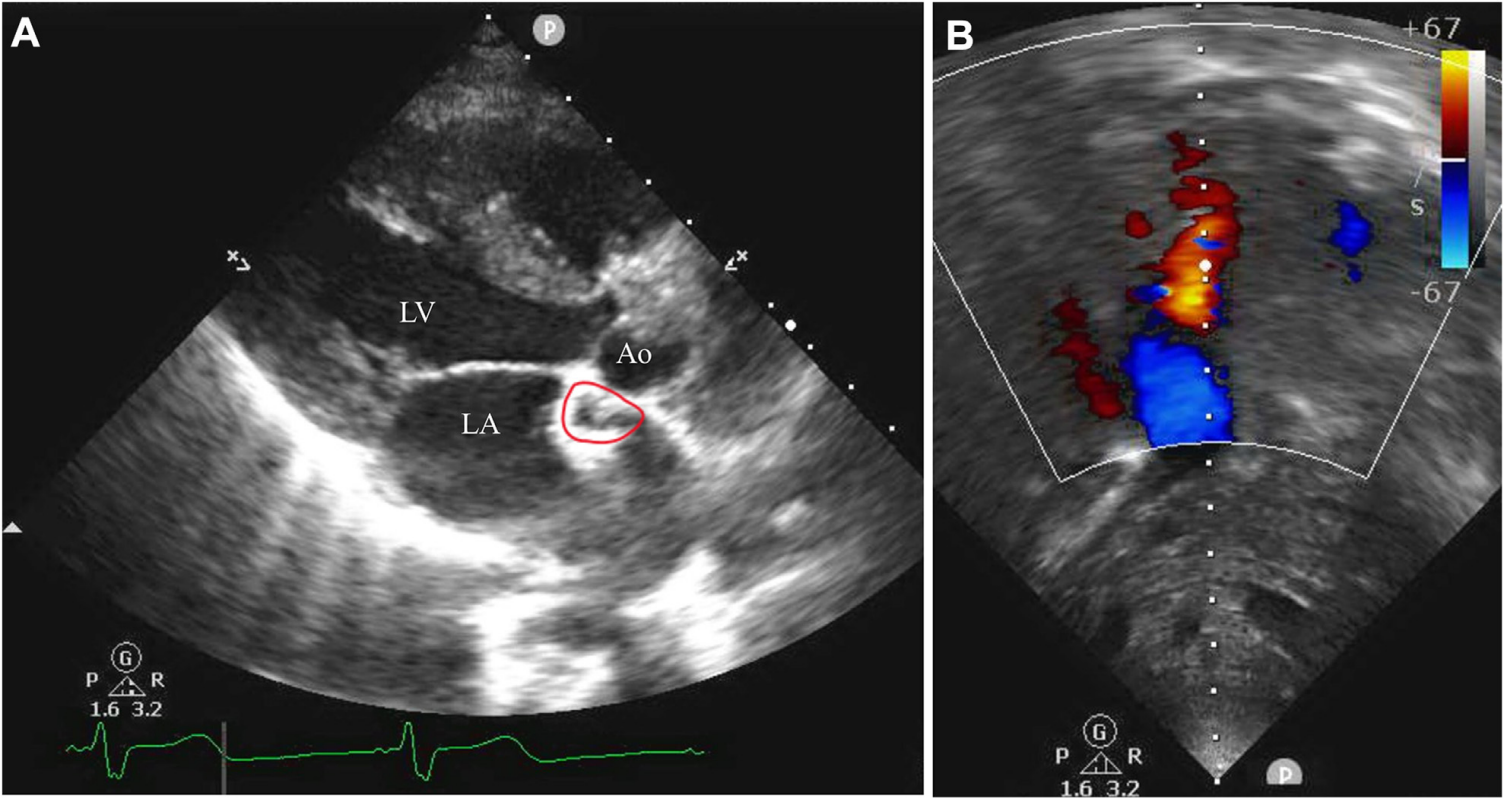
Echocardiogram demonstrating patency of left superior vena cava–right atrial baffle in patient 12. (A) Parasternal long-axis view demonstrating atrial baffle (red circle) near the superior aspect of the left atrium. (B) Doppler ultrasound demonstrating flow through the patent atrial baffle. (Ao, aorta; LA, left atrium; LV, left ventricle.)
